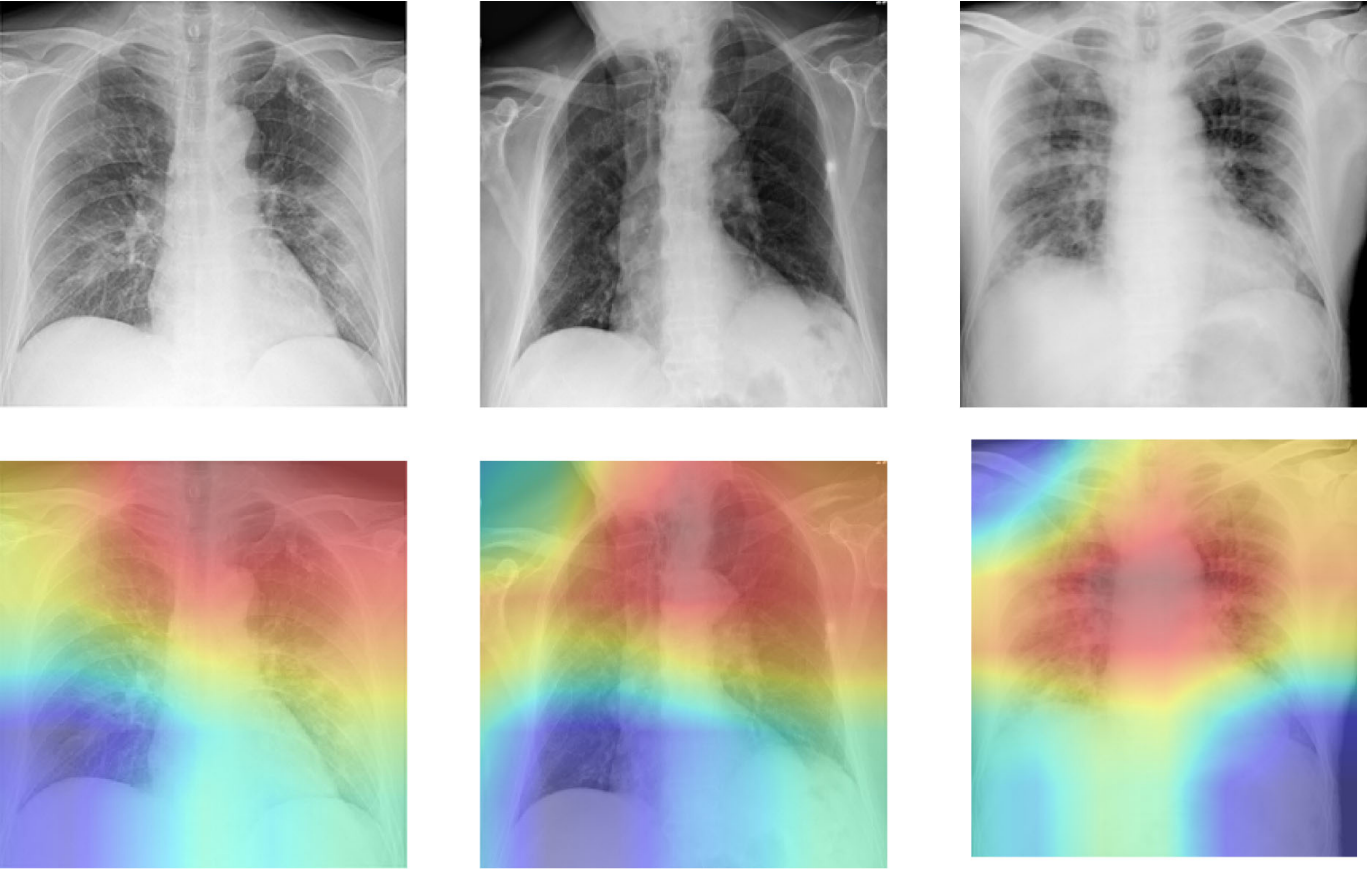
Saliency maps of some testing images for the classification of COVID-19 vs. healthy and pneumonia X-ray images. Red areas show which parts of the image the algorithm believes to be useful in the diagnosis.
